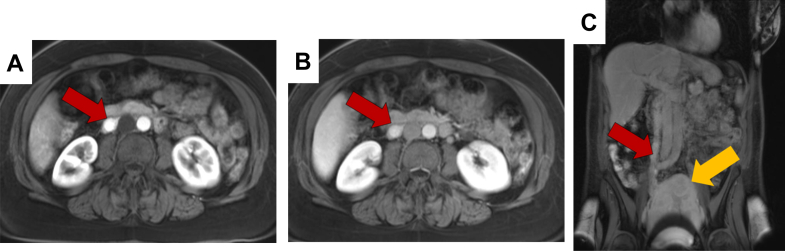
MRI in Arterial, Venous, and Delayed Postcontrast Phases MRI axial (A) arterial and (B) venous postcontrast phase images show an enhancing intraluminal mass (red arrows) within the IVC. (C) Coronal delayed postcontrast phase image demonstrates a heterogeneously enhancing lesion extending along the IVC and contiguous with the right gonadal vein. Persistent enhancement throughout the mass indicates high vascularity, consistent with a fibroid origin. The red arrow marks the intravascular tumor component, and the yellow arrow identifies the uterine leiomyoma. CT = computed tomography; IVC = inferior vena cava; MRI = magnetic resonance imaging; RA = right atrium.
